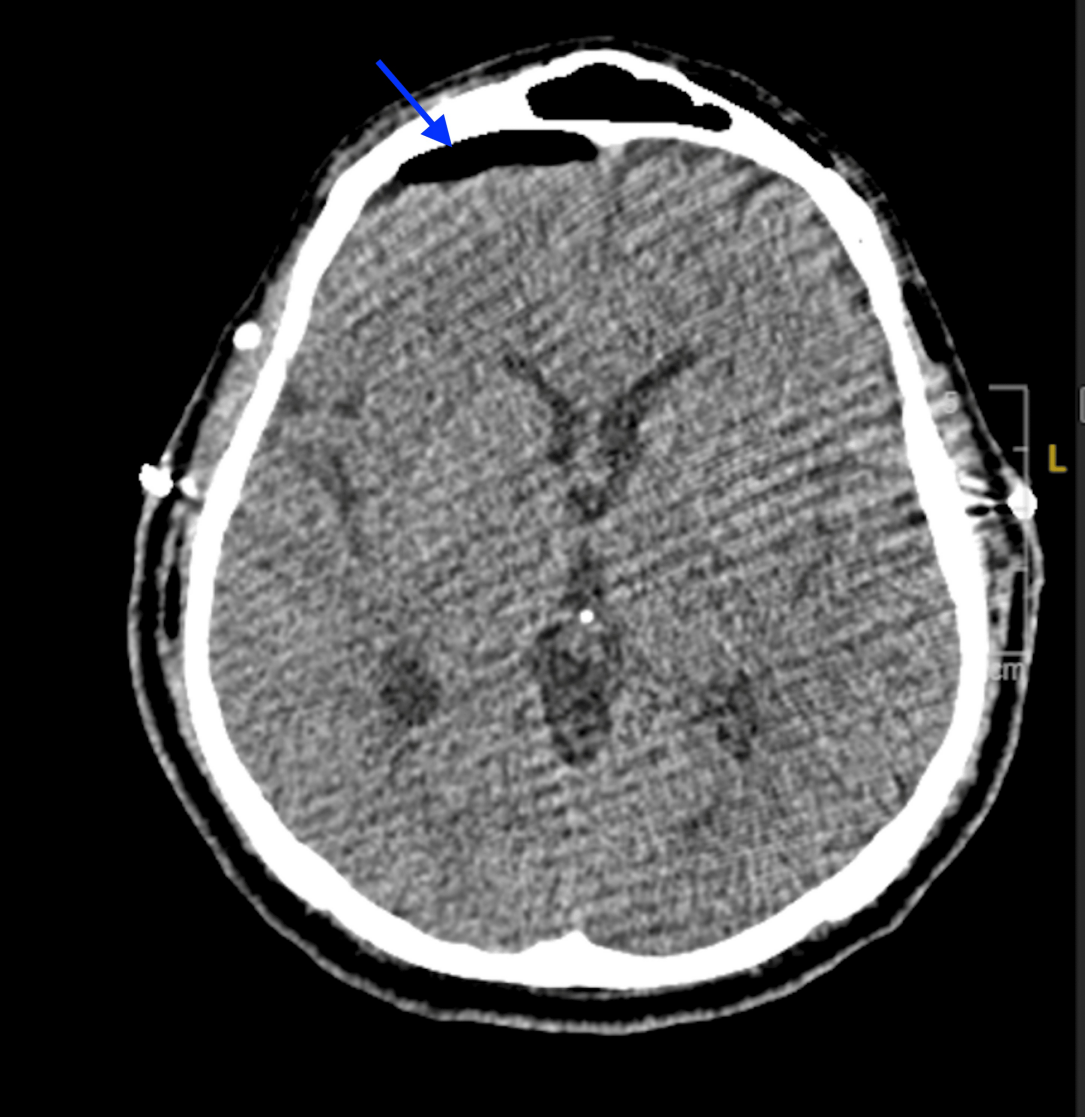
Axial CT head without contrast on postoperative day 4 The image demonstrates a new right frontal pneumocephalus (arrow), suggesting CSF leak with air entry CSF: cerebrospinal fluid; CT: computed tomography
